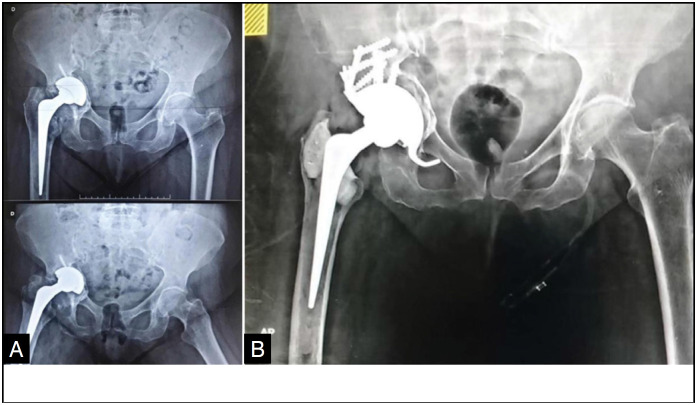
Case 01 (Image A: Preoperative X-ray and image B: X-ray three months after surgery).
